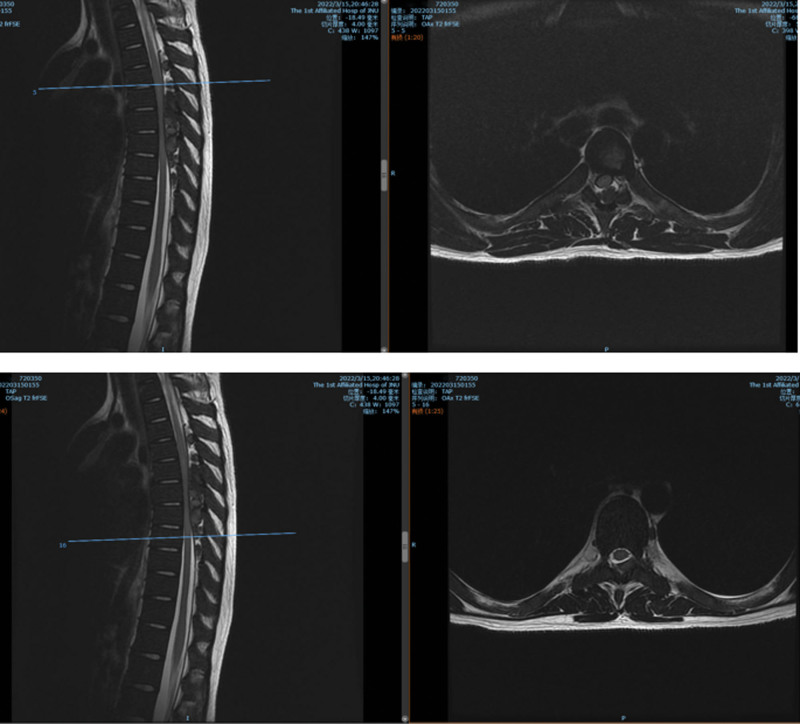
Computed tomography (CT) bone window.
